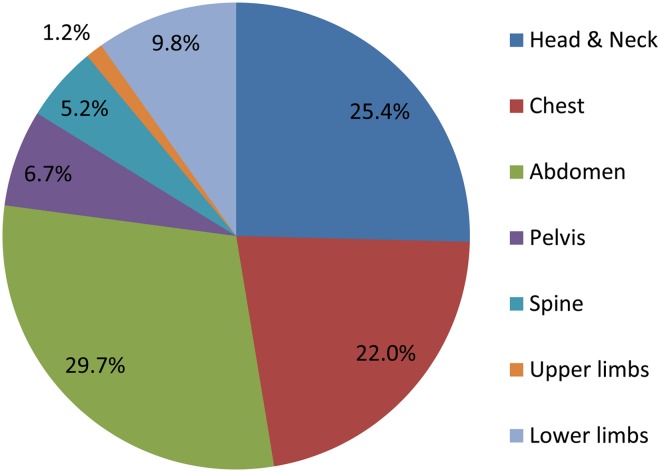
Distribution of CT examinations among the various regions of the body.
